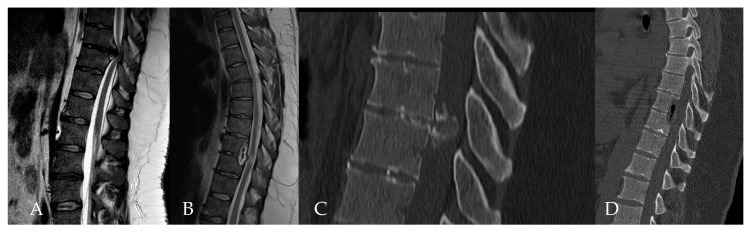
Patient No. 4 (A) Preoperative and (B) postoperative sagittal T2-weighted MRI of the thoracic spine with (C) preoperative and (D) postoperative sagittal CT of the thoracic spine following complete resection of the Th9/10 disc.
